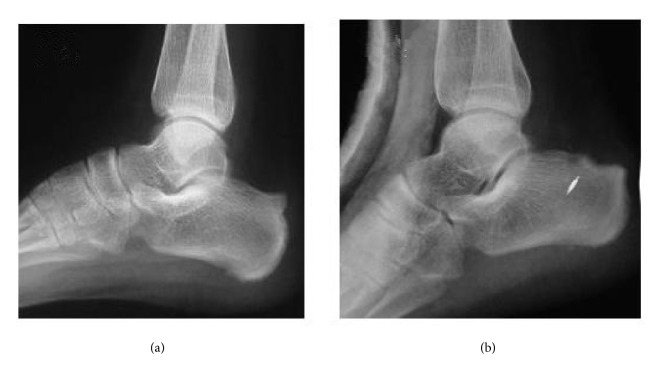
The standing lateral foot X-ray preoperatively (a) and postoperatively (b) showed the calcaneal prominence was excised.
